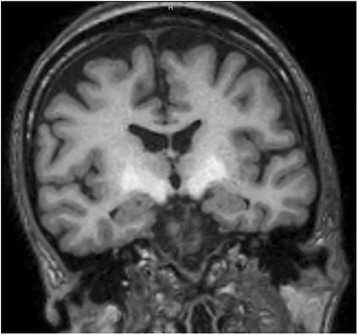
BG-MnIL MRI image. T1 sequence Brain MRI showing hyperintensity signal in the basal ganglia as consequence of manganese deposition
